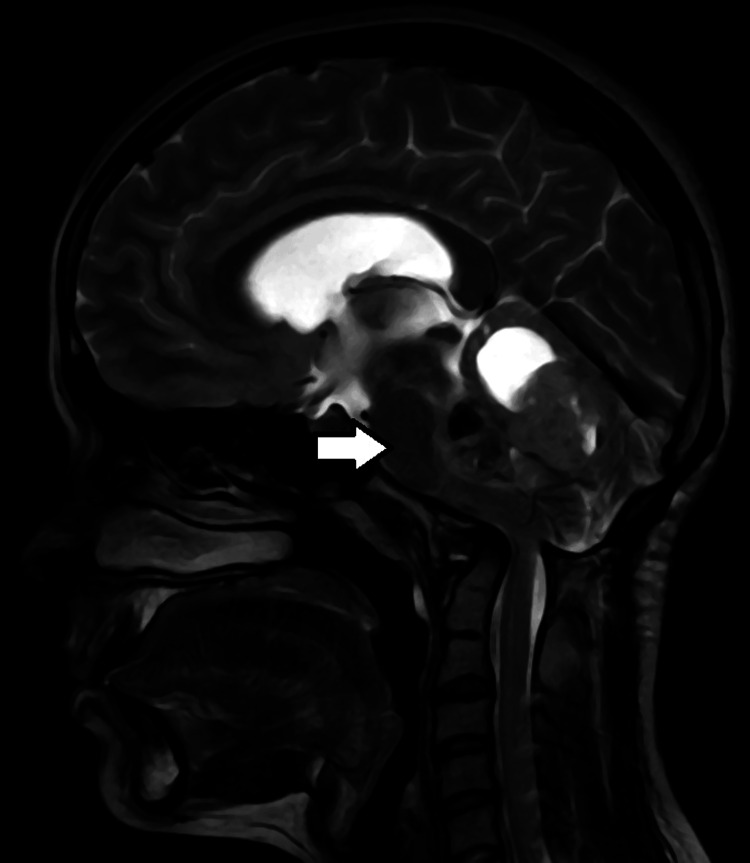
Sagittal T2 MRI brain image The white arrow shows the mass effect of the lesion in the form of anterior displacement of the brain stem. MRI: Magnetic resonance imaging
